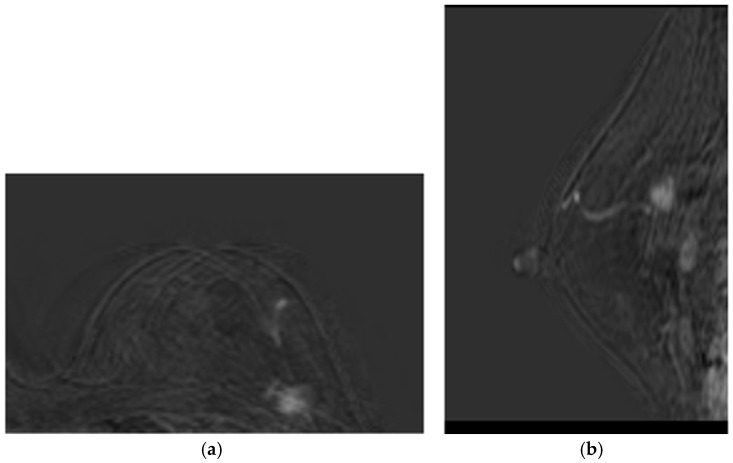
Post-NAC CE-MRI of Patient 1: (a) sample axial projection; (b) sample sagittal projection. The lighter areas, on the gray/black background, depict the tumoral ROIs.
